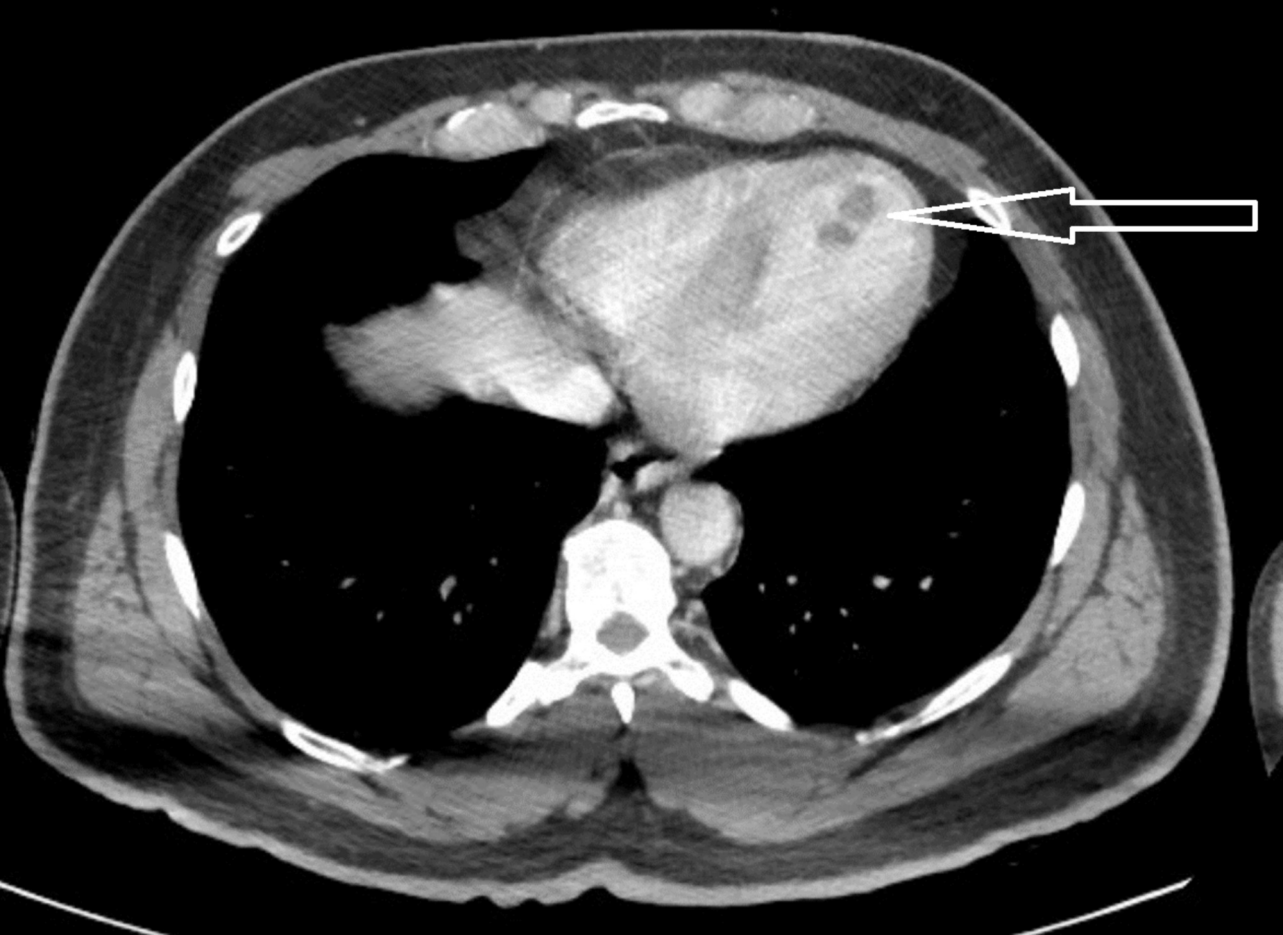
CT demonstrating intraventricular cardiac thrombus (arrow)
